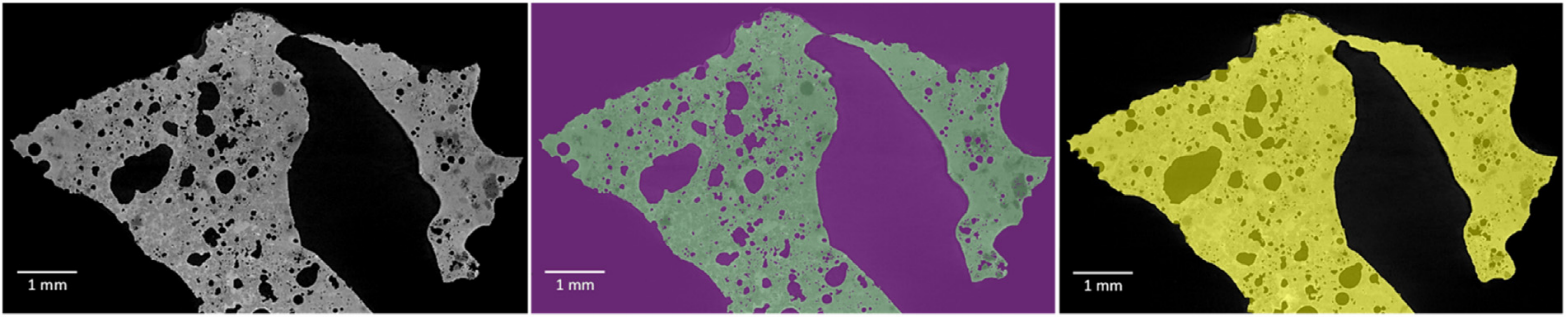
An ortho slice with grey scale according to X-ray attenuation intensities to the left, the segmented model in the middle with the ROI “Ash Particle” as green and ROI “Air” as violet, and to the right a ROI “Filled particle” in yellow.
